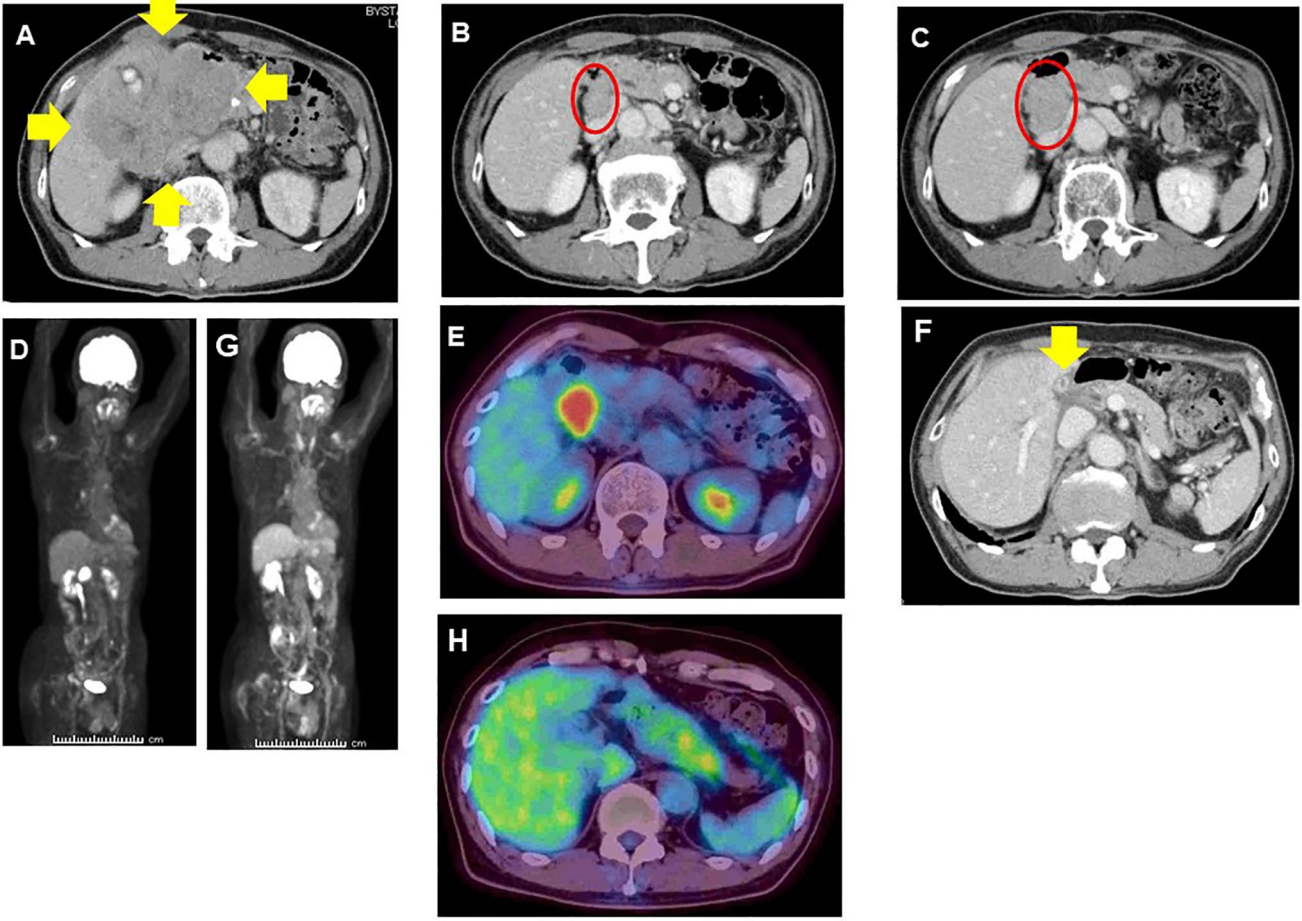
Enhanced computed tomography (CT) and fluorodeoxyglucose-positron emission tomography-computed tomography (FDG-PET/CT) images. Enhanced CT showing a gallbladder mass before treatment (a). The tumor size was about 130 mm. Two high-density spots revealed gallbladder stones (yellow arrowhead) and a high-density dot was bile duct plastic stent for biliary drainage (yellow arrow). Two cycles of chemotherapy resulted in a partial response with remarkable tumor shrinkage (b). After four cycles chemotherapy, tumor response was continuing (c). After five cycles of chemotherapy, the primary huge tumor lesions disappeared and distal migration of the plastic stent (d). However, the hepatic lymph node surrounding the hepatic hilum was slightly enlarged, about 18 mm (yellow arrowhead). After seven cycles of chemotherapy, the lymph node was remarkably enlarged, about 28 mm (e) (yellow arrowhead). Maximum intensity projection (MIP) (f) and FDG-PET/CT imaging (g) before chemoradiotherapy revealed uptake in the portal lymph node (SUV max 8.2) (yellow arrowhead). After chemoradiotherapy, the enlarged hepatic lymph node shrinked, and the CT value of panniculitis around the gallbladder slightly increased (h) (yellow arrowhead). MIP (i) and FDG-PET/CT imaging (j) after chemoradiotherapy did not reveal increased uptake in the gallbladder and lymph node
